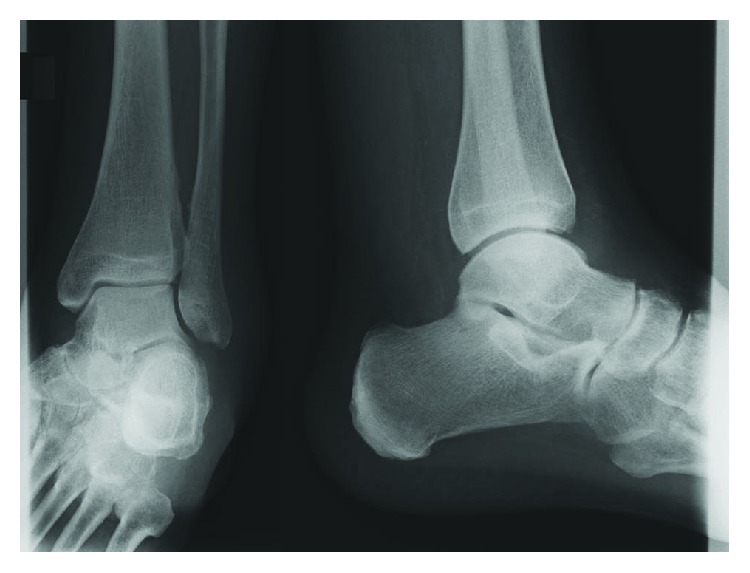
Preoperative anterior-posterior and lateral radiographs of the left ankle joint showing no signs of any osseous destruction or joint damage.
